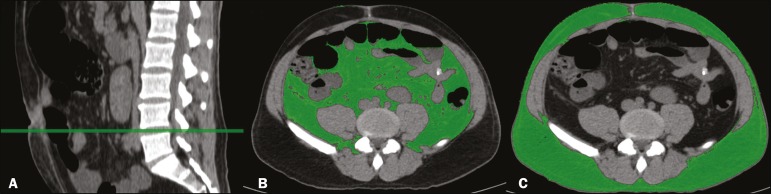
CT measurements of visceral and subcutaneous fat. A: Sagittal plane used in order to set the axial plane between the fourth and fifth lumbar vertebral bodies. B,C: Segmentation of visceral and subcutaneous fat (B and C, respectively) at the same level.
